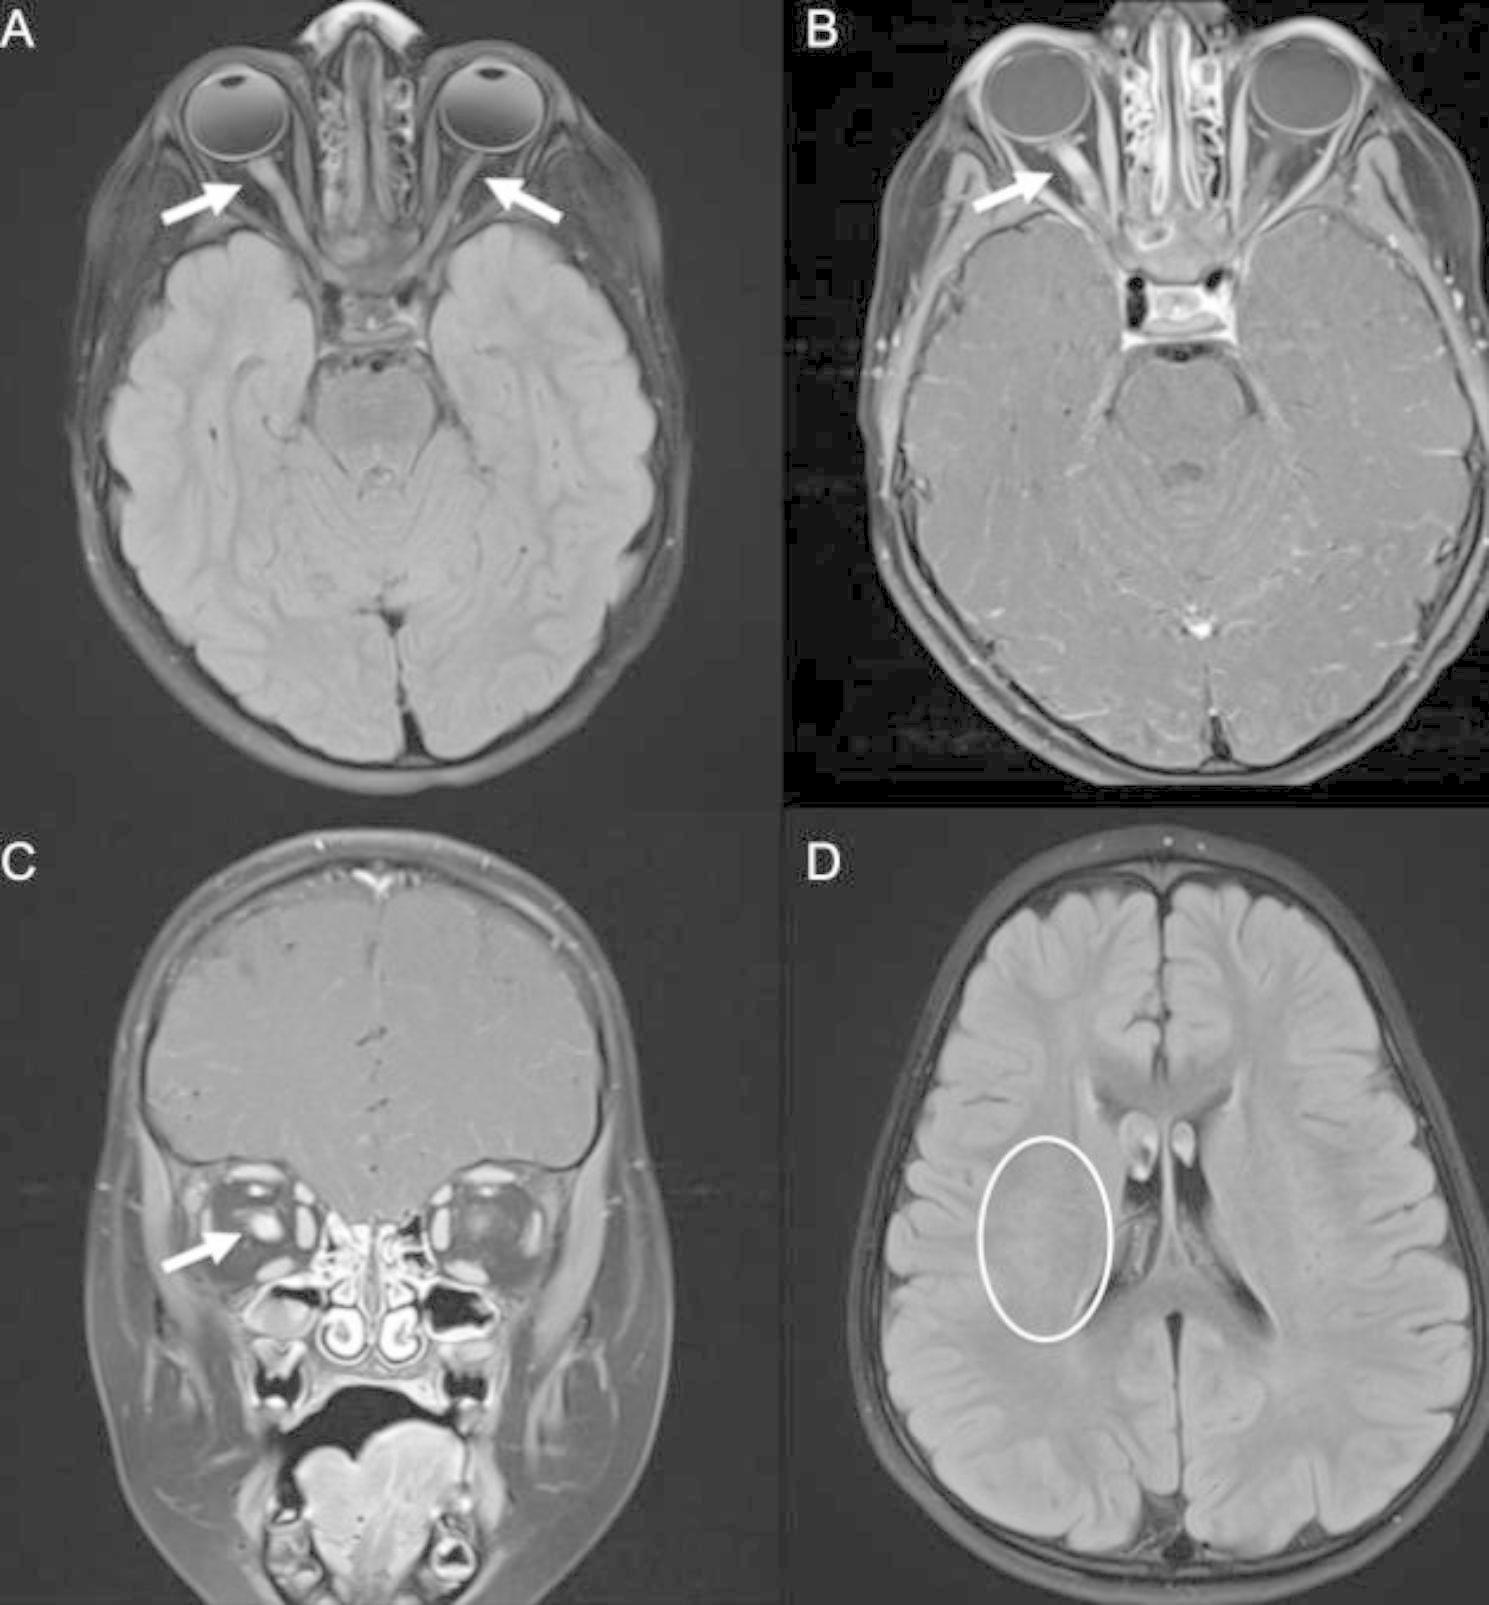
MRI brain images from second hospitalization. Axial FLAIR sequence demonstrating (A) T2/FLAIR hyperintensity of bilateral optic nerves right > left, optic chiasm, and optic tracts with (B) corresponding T1 gadolinium enhancement, indicating relapsed bilateral optic neuritis. (C) T1 gadolinium enhancement of optic nerves right > left also noted in coronal images. (D) Previously noted T2/FLAIR hyperintensities resolved
